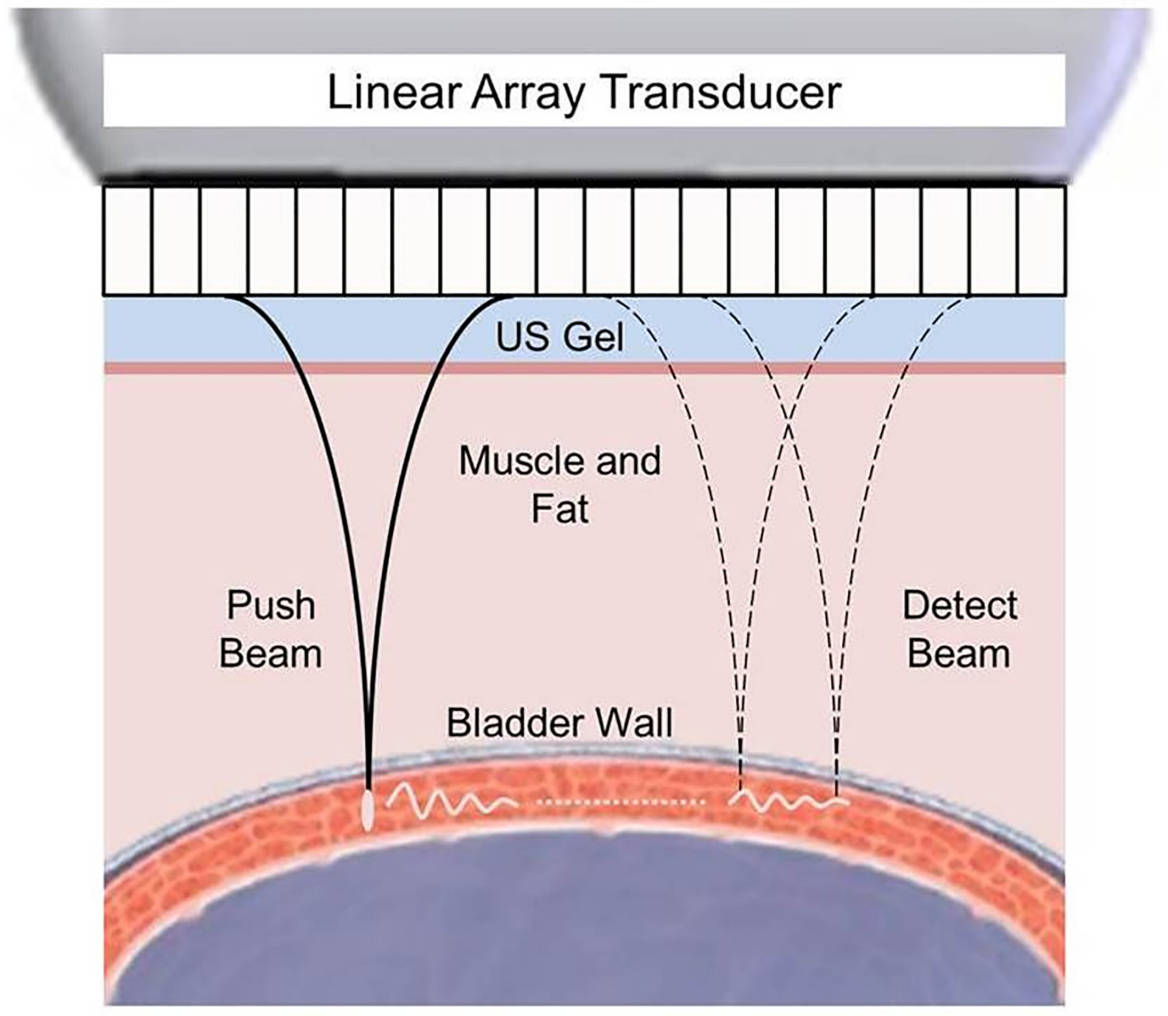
An ultrasound array transducer is utilized to generate an ARF beam (push beam) on the bladder wall, generating an anti-symmetric Lamb wave displacement field. The displacement field is tracked by a pulse-echo detection beam (detect beam). In order to identify the bladder wall, a line is drawn along the bladder wall on the B-mode image. The displacement field is measured along this line. To obtain appropriate coupling between the transducer and skin surface above the bladder, standard ultrasound coupling gel (US gel) is utilized.
